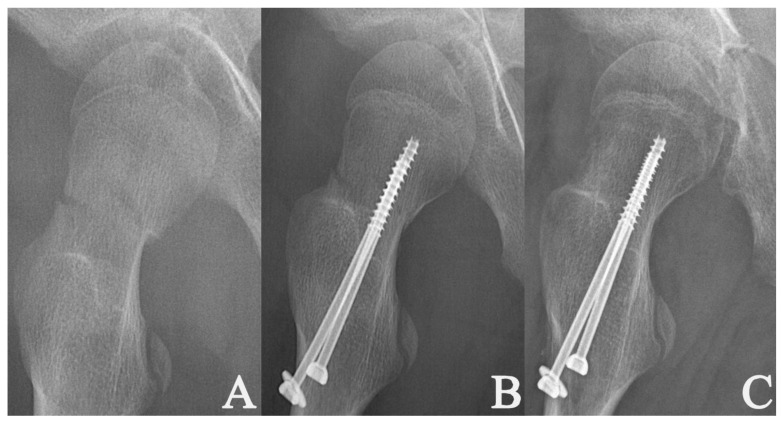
Radiographs of a 9-year-old girl with a displaced Delbet–Colonna type II fracture [15] (A); radiographs after closed reduction and internal fixation with two cannulated screws filling 11.9% of the femoral neck; the fracture was partially compressed by the hardware (B); the radiological healing at 1.9 months post-surgery (C).
